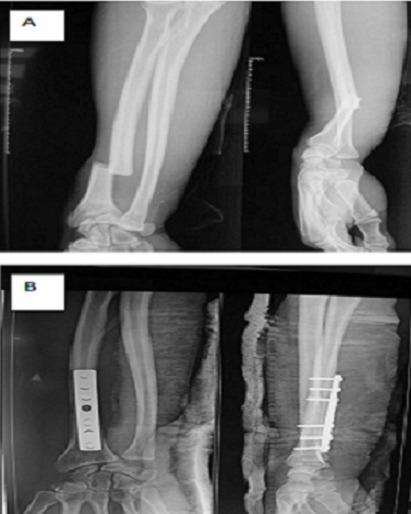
A) Radiographies du poignet (face et profil) montrant une fracture de Galeazzi type 3; B) Radiographies du poignet (face et profil) après ostéosynthèse de la fracture du radius par plaque vissée DCP 07 trous avec réduction spontanée de la luxation radio-ulnaire distale
